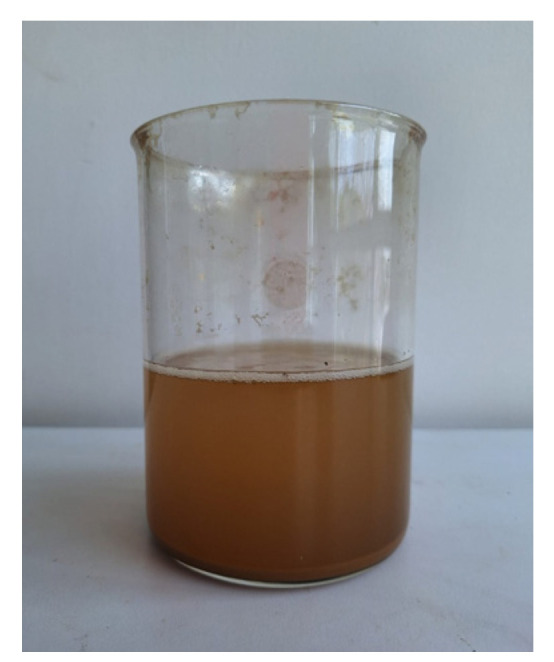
GO-DCNS dispersed in water by ultrasonication.
